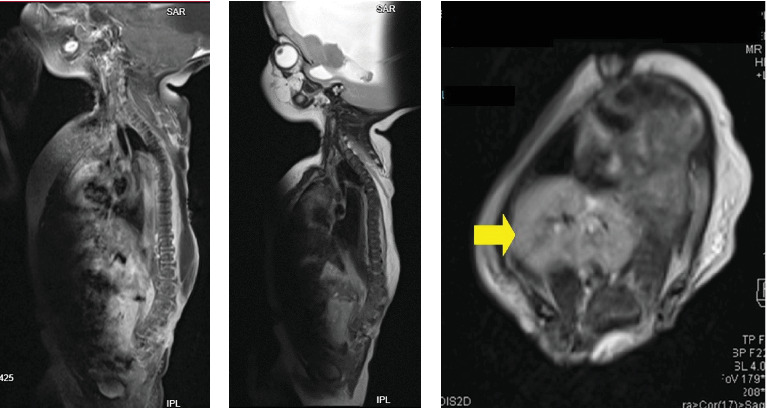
MRI spine and abdomen showing open defect in the back with absent posterior elements of the spine from the level of T4 with a short spinal cord seen ending at T6 level (A, B). The kidneys appear fused and seen in the right iliac region in location (C, yellow arrow). Features suggestive of severe hydrocephalus with corpus callosum agenesis and schizencephaly, spina bifida, and renal fusion.
